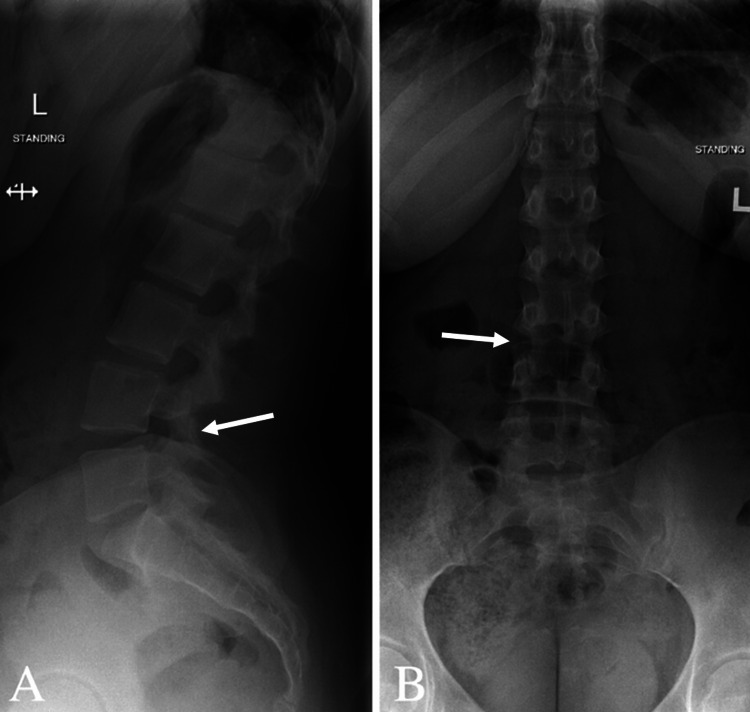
Radiograph of the lumbar spine (A) lateral view and (B) anterior-posterior view demonstrating five lumbar vertebrae. Maintained vertebral body heights and disc spaces. No spondylolisthesis. Patent neural foramina and minimal levocurvature of the lumbar spine.
